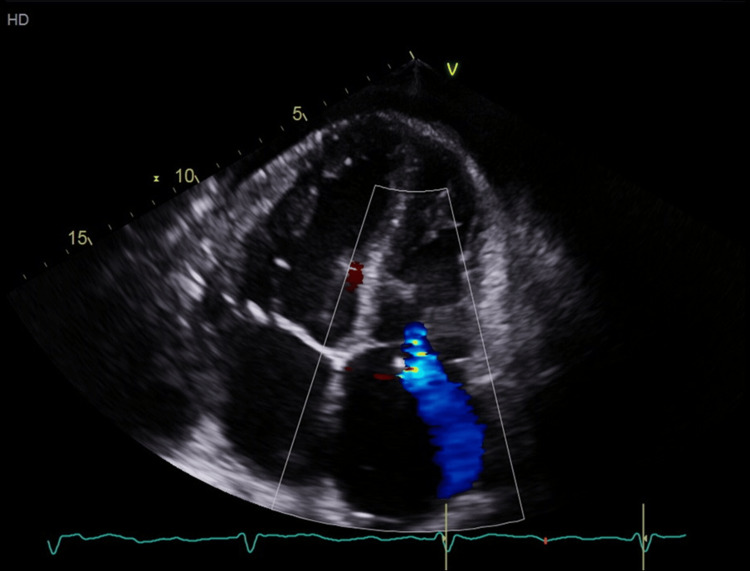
Transthoracic echocardiogram eight months after pacemaker upgrade and 18 months from initial pacemaker placement showing a reduction in tricuspid regurgitation to a grade of mild to moderate. By Mayo Clinic convention, the left ventricle is inverted on the image (left upper cardiac chamber on image).
